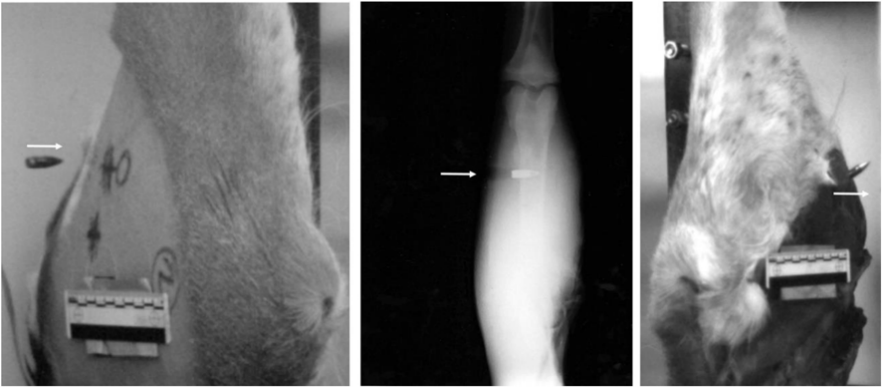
Arrow indicates projectile direction of travel—left: oblique view of front face of deer limb with 7.62 mm projectile about to strike symmetrically; middle: flash X-ray imaging demonstrating 7.62 mm projectile travelling through suspended deer limb, yawing slightly; right: oblique view of rear face of deer limb with 7.62 mm projectile exiting deer limb, yawing significantly
